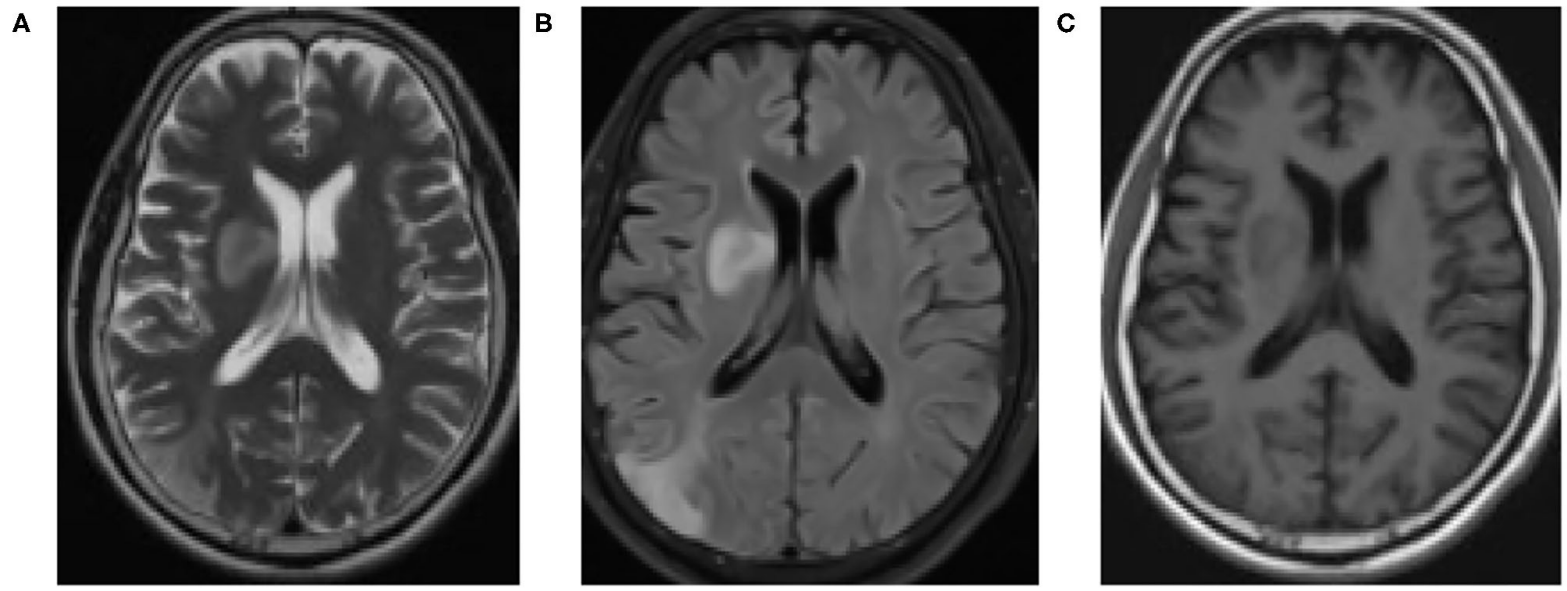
MRI examination results. (A) before drug treatment; (B) during drug treatment; (C) one week after drug discontinuation.
